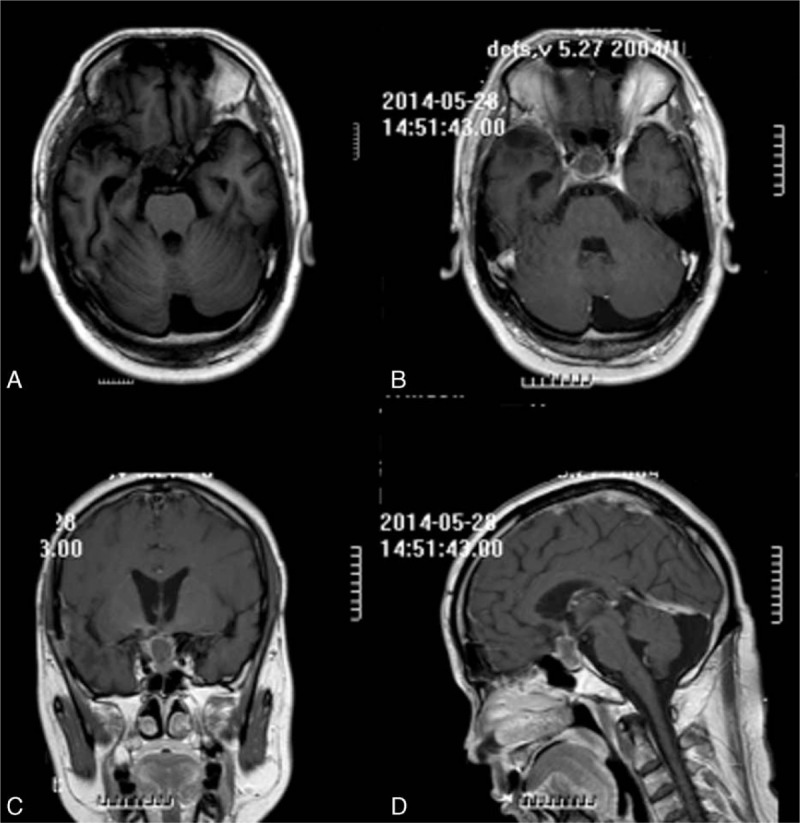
Magnetic resonance imaging findings revealed that the tumor size was 1.7 cm × 1.4 cm × 2.1 cm after Chinese herbal decoction combined with brucea soft capsules) (May 2014) (A–D).
